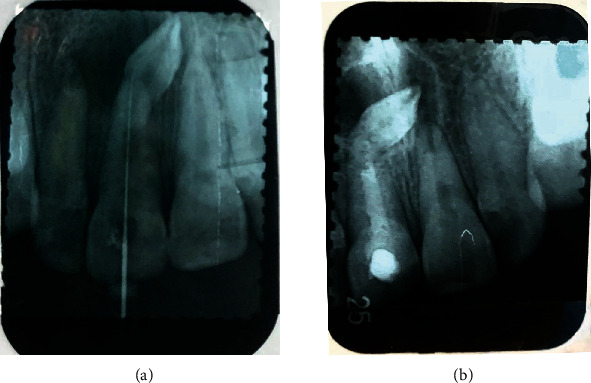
Periapical radiograph: (a) #11 with open apex; (b) one year later found apexification material on root canal and closed apex. The impacted mesiodens shown surrounded with cyst between apical of #21 and #11.
